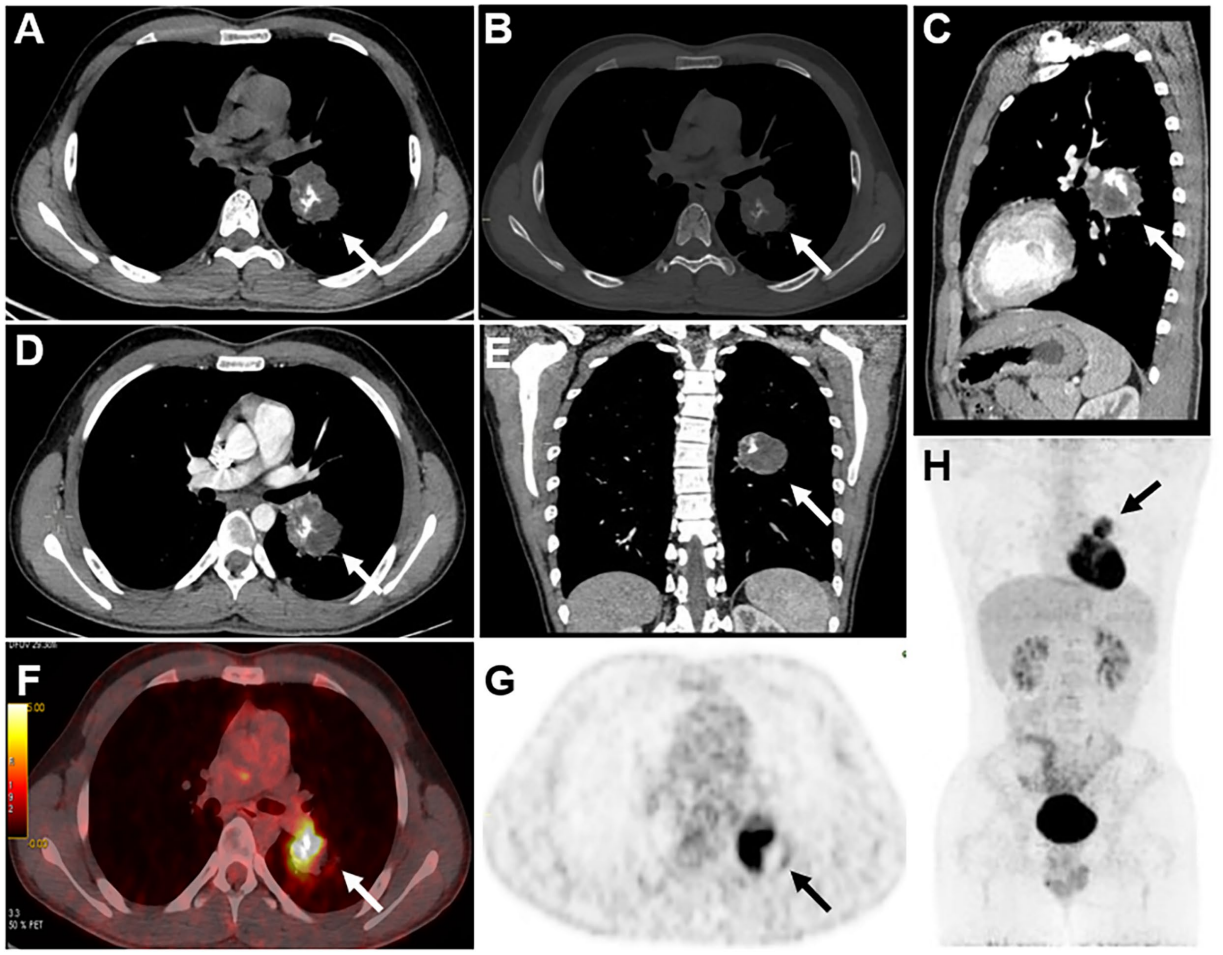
CT scans of the mediastinal window (A), bone window (B), and sagittal mediastinal window (C) revealed a mass-like soft tissue density shadow in the dorsal segment of the lower lobe of the left lung. The mass had an irregular sphere-like margin, uneven density within the mass, and irregular eccentric ossification or calcification. In addition, axial (D) and coronal (E) enhanced CT images also revealed a heterogeneous mass with no enhancement in the cystic portion. ^18^F-FDG PET/CT revealed uneven increased metabolism of the mass in the lower lobe of the left lung, with barely absent uptake in its postero-lateral side (F,G), and the area in the postero-lateral side with increased metabolic activity in lymph nodes was also observed, which also appears hypodense on contrast-enhanced CT. No elevated metabolic activity was observed in other areas on the PET/CT scan (H). Based on a comprehensive evaluation of CT and PET/CT findings, the initial staging before surgery was T2N1M0.
